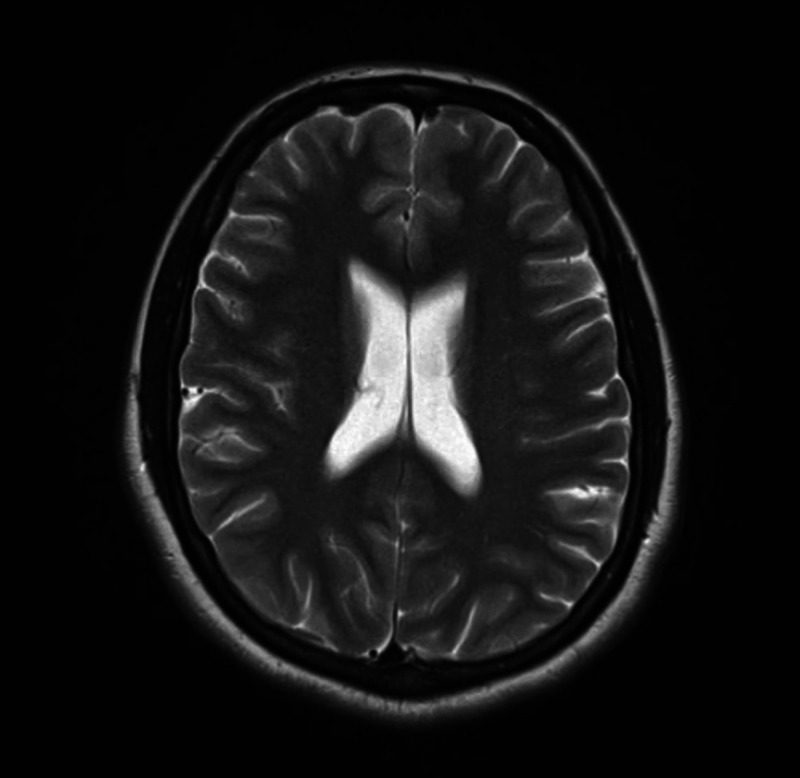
Patient's normal MRI cranium No ventriculomegaly was seen. No focal, hyperdense/hypodense lesions were seen.
